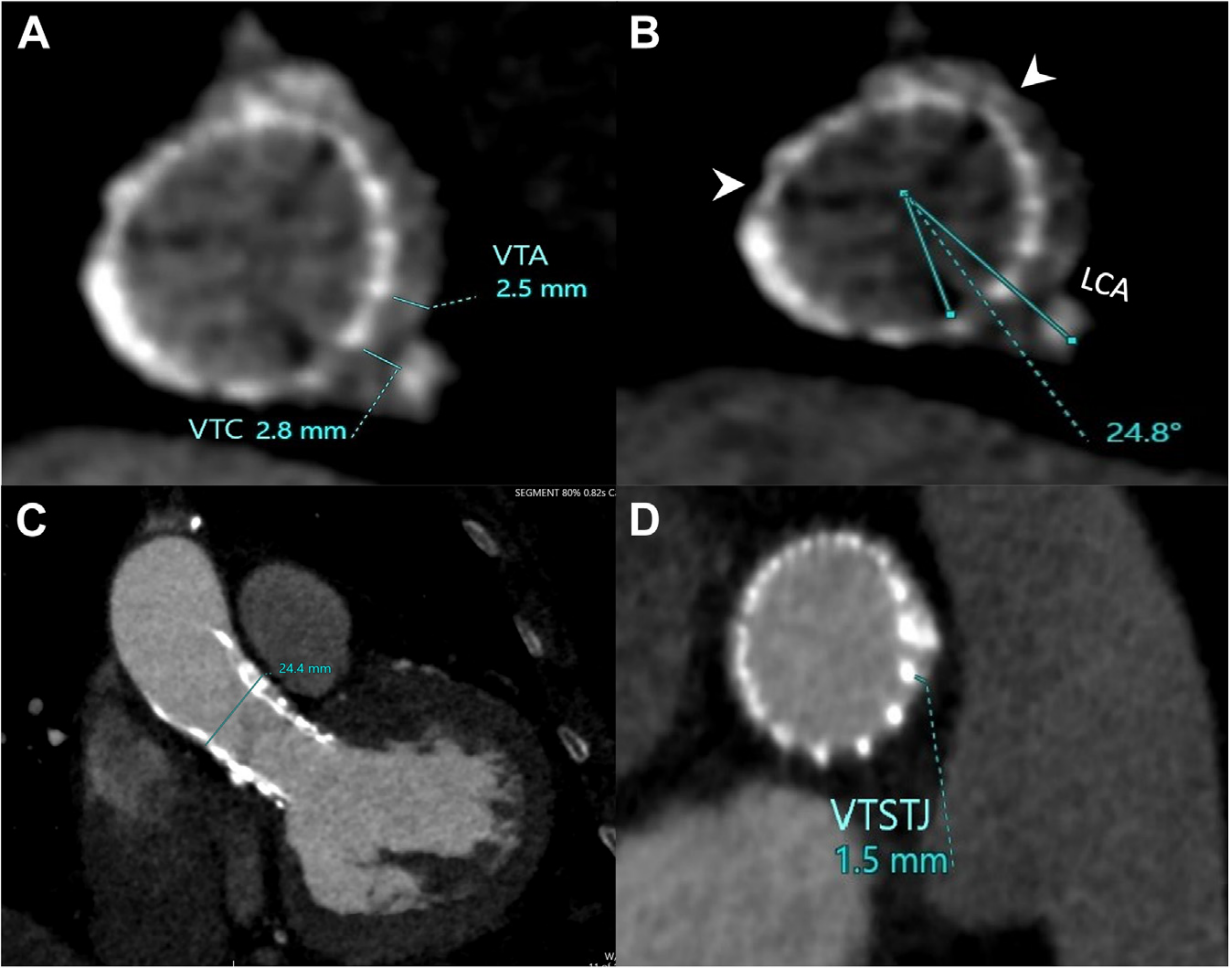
Multidetector Computed Tomography–Driven Measurements (A) The Evolut PRO (Medtronic)–to-aorta distance is 2.5 mm, the Evolut PRO–to-coronary distance is 2.8 mm, (B) the commissural-coronary angle measures 24.8° (arrowheads highlight the neocommissures), (C) the sinotubular junction width is 24.4mm, and (D) the valve-to-sinotubular junction (VTSTJ) distance is 1.5 mm. VTA = valve to aorta; VTC = valve to coronary.3.Planning the PCI procedure: The LCA origin was detected at the midlevel of the fourth Evolut node, 1 node above the upper border of the SAPIEN metallic frame. MDCT analysis was used to determine the optimal projection for LM artery cannulation, which was confirmed to be left anterior oblique (LAO) 40° with a cranial angulation of 15° (Figures 5A and 5B).
